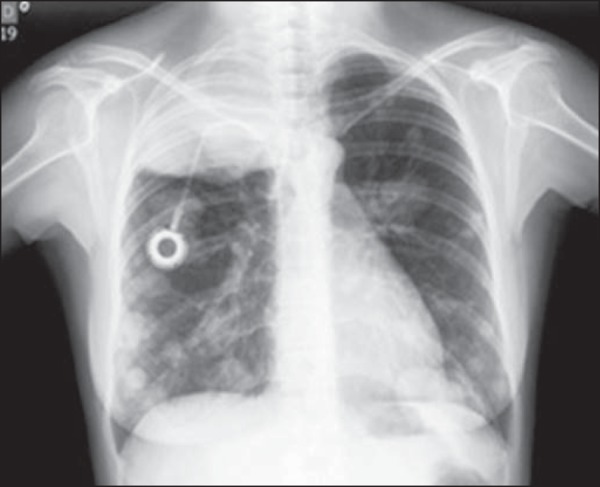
Posteroanterior chest X-ray, acquired during follow-up, showing
